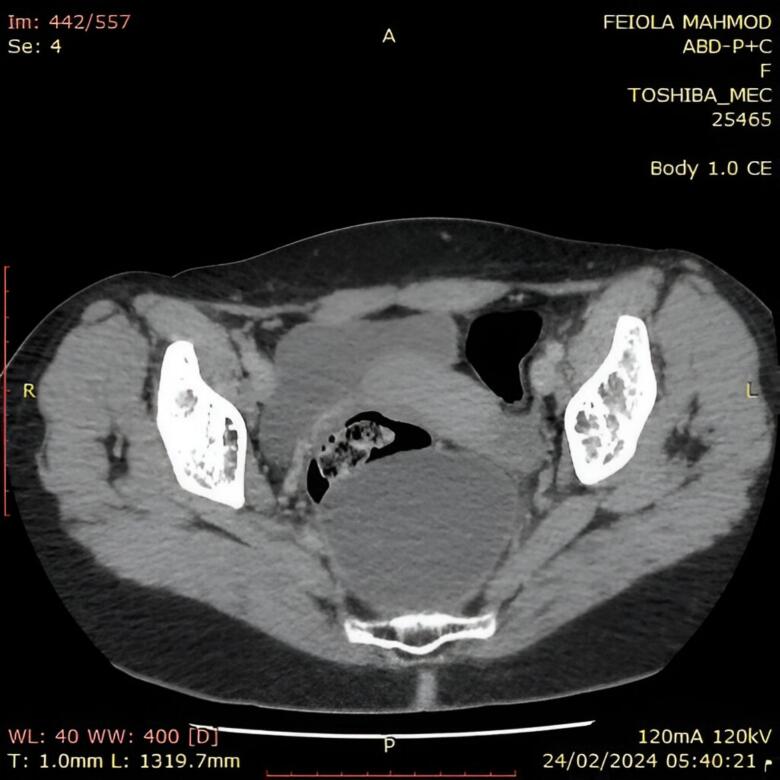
Computed tomography of presacral teratomas. Axial (A) and sagittal (B) sections. “A” Axial image of contrast enhanced computed tomography of abdominal and pelvis showing a large cystic mass near the back edge of the middle and lower portions of the rectum. “B” Sagittal image of abdominal ultrasound showing a large cyst behind the cervix.
